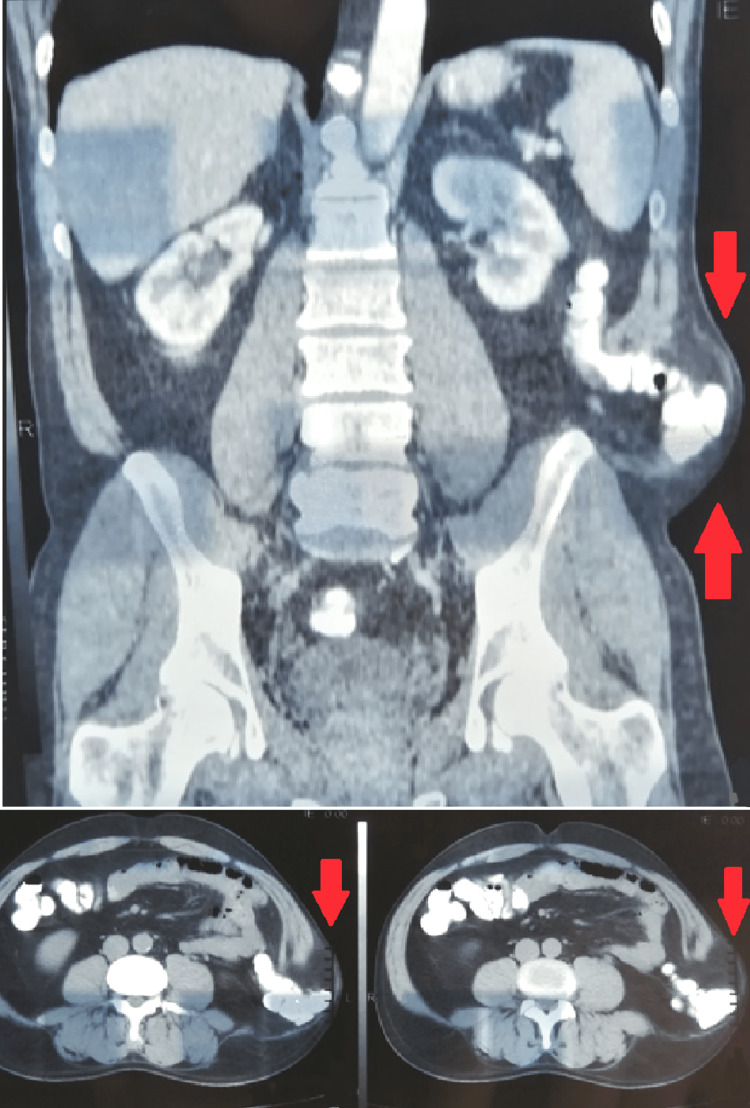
Abdominal computed tomography scan CT scan of the abdomen revealing the presence of a defect in the left lateral abdominal wall, which was 9 cm in diameter, and a hernia sac containing loops of the large bowel.
